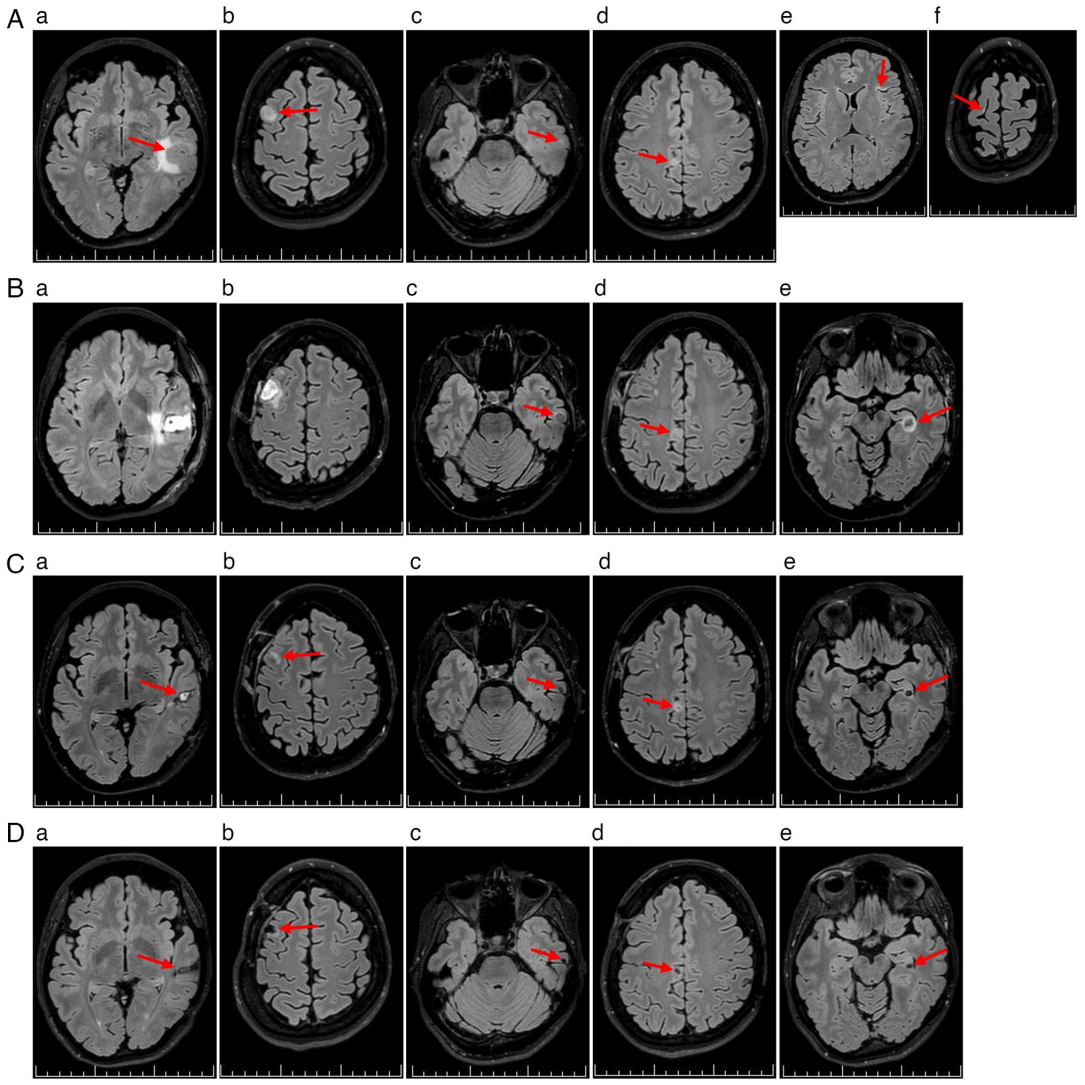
Timeline of the radiological course (MRI fluid-attenuated inversion recovery sequences). (Aa-Ad) Initial images showing four malignant melanoma brain metastases (red arrows). (Ae and Af) Images showing two suspected brain metastases (red arrows). (Ba and Bb) MRI 2 days after surgical resections. (Bc and Bd) Enlargement of the two remaining metastases (red arrows). (Be) Growth of a new metastasis in the dominant hippocampal region (red arrow). (Ca and Cb) Favorable postoperative changes (red arrows). (Cc-Ce) Notable reduction in metastatic lesion volume (red arrows). (Da and Db) Further favorable postoperative changes without signs of recurrence (red arrows). (Dc-De) Partial disease remission (red arrows). Scale bars, 10 mm.
